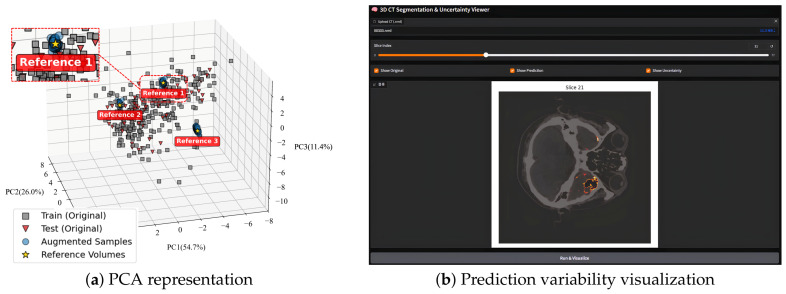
PCA-based visualization of CSRA-augmented samples and example prediction variability visualization interface. (a) Three-dimensional landmark-based PCA projection of original training samples, original test samples, CSRA-augmented samples, and selected reference volumes. The axes correspond to PC1, PC2, and PC3, explaining 54.7%, 26.0%, and 11.4% of the landmark-space variance, respectively, with a cumulative explained variance of 92.0%. Enlarged dashed regions highlight representative reference-centered areas in the PCA space for qualitative visualization of CSRA-induced geometric variation. (b) Example interface for visualizing CT slices, predicted hemorrhage regions, and qualitative prediction variability maps obtained from multiple reference-based CSRA predictions.
